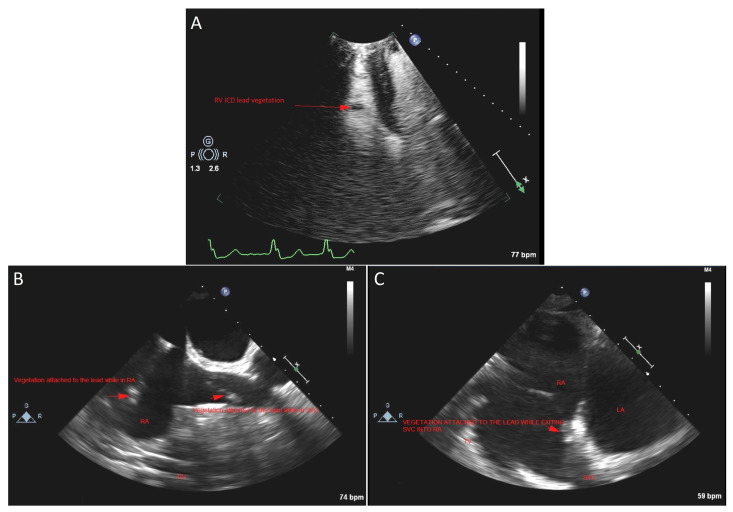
(A) Transthoracic echocardiogram (TTE) view that shows right ventricle (RV) ICD lead vegetation. (B) Transesophageal echocardiogram (TEE) view that shows vegetation attached to the ICD lead in the superior vena cava (SVC), but also vegetation attached to the lead while in the right atrium (RA). (C) TEE view that shows a small mass attached to the ICD lead while exiting the SVC into the RA, suspicious for vegetation. LA: left atrium.
